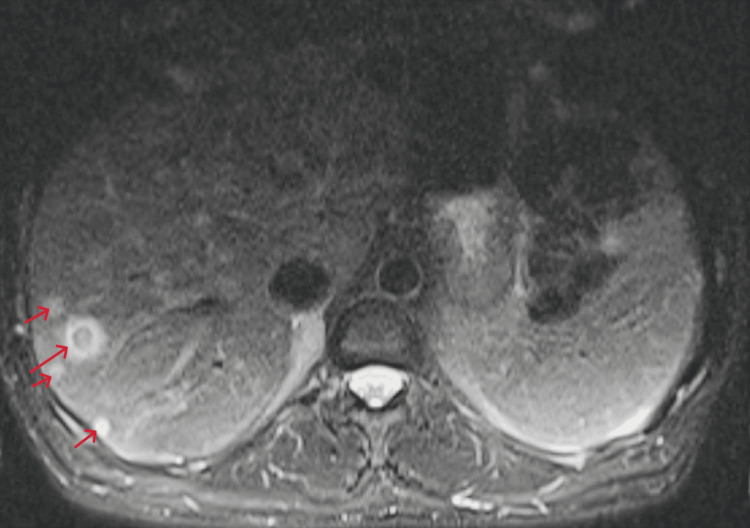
Abdominal MRI with and without contrast showing innumerable T2-hyperintense lesions demonstrating a halo sign, rim enhancement, and central diffusion restriction, concerning for micro-abscesses
